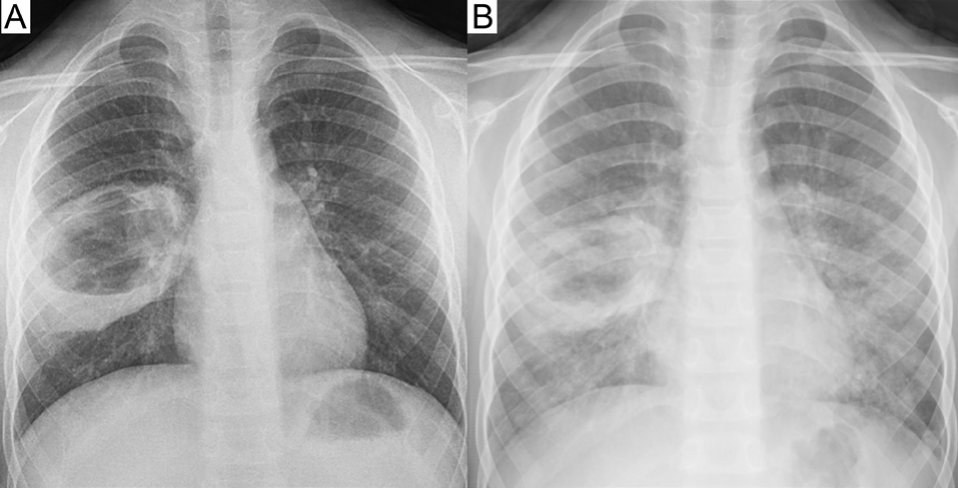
In a 7-year-old male who presented with cough and hydatoptysis, chest x-ray (a) taken at the time of admission and chest x-ray showing bilateral diffuse pneumonia 2 days after the perforation (b) are seen.
